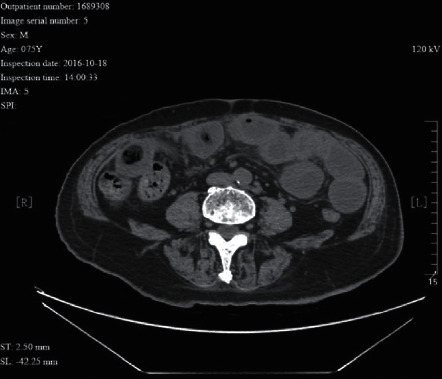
A 75 year-old male patient with adhesive intestinal obstruction complicated with partial small intestinal volvulus. He had received “radical resection of rectal cancer”. The CT image shows bowel wall thickening proximal to the obstruction. During surgery, bowel wall ischemia was seen at the site of severe adhesion.
